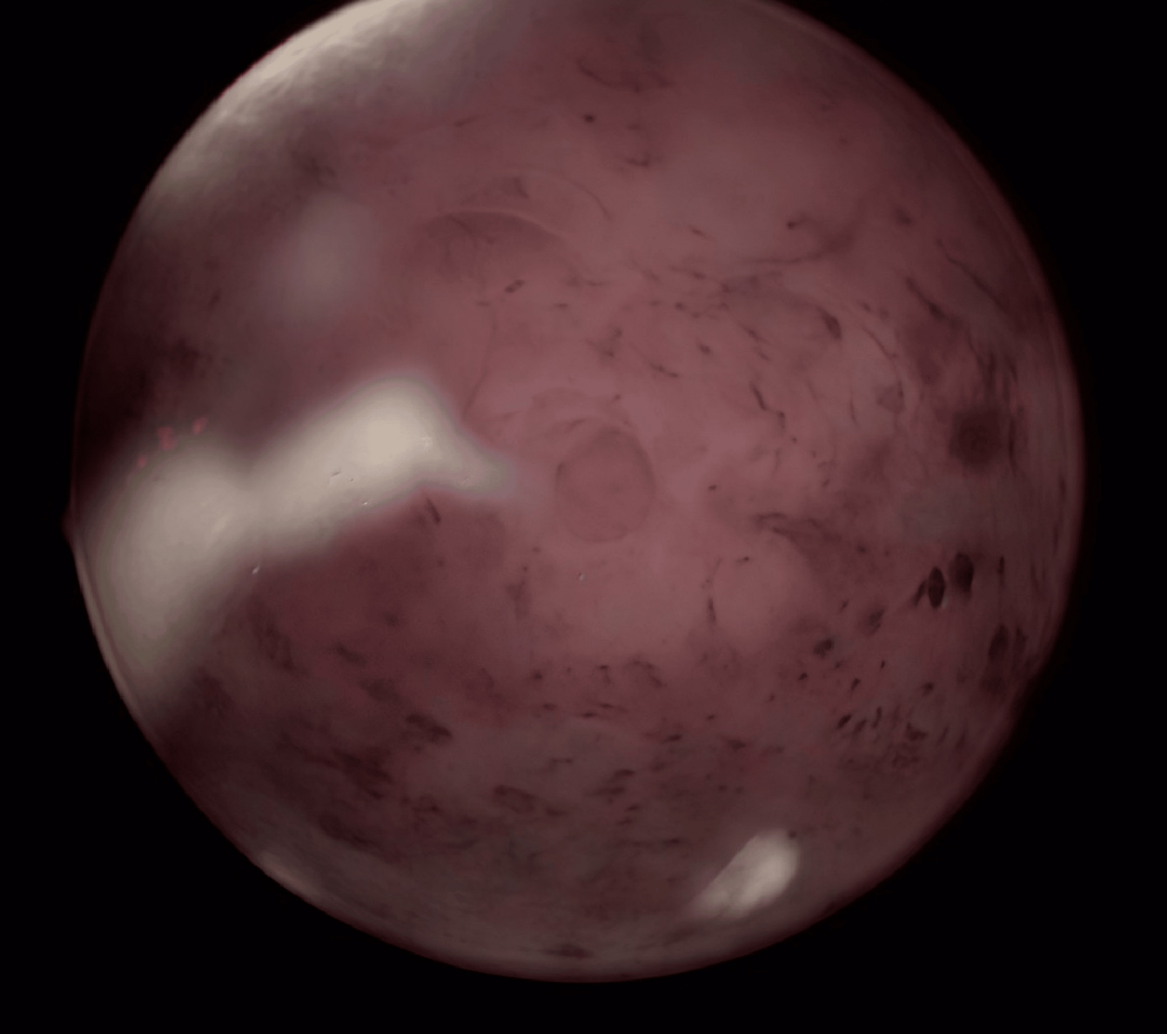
Uterine cavity exhibiting vascular dystrophy Ostial region hysteroscopic view of the uterine cavity showing widespread tortuous and dilated vascular channels distributed over the fundal and posterior walls. The image was captured during diagnostic hysteroscopy in a patient with a history of MR-guided high-intensity focused ultrasound (HIFU) treatment for fibroids. The diffuse vascular distortion and irregular endometrial texture demonstrate endometrial vascular dystrophy coexisting with leiomyomatous changes. The findings emphasize the role of prior uterine interventions and altered angiogenesis in EVD pathogenesis.
